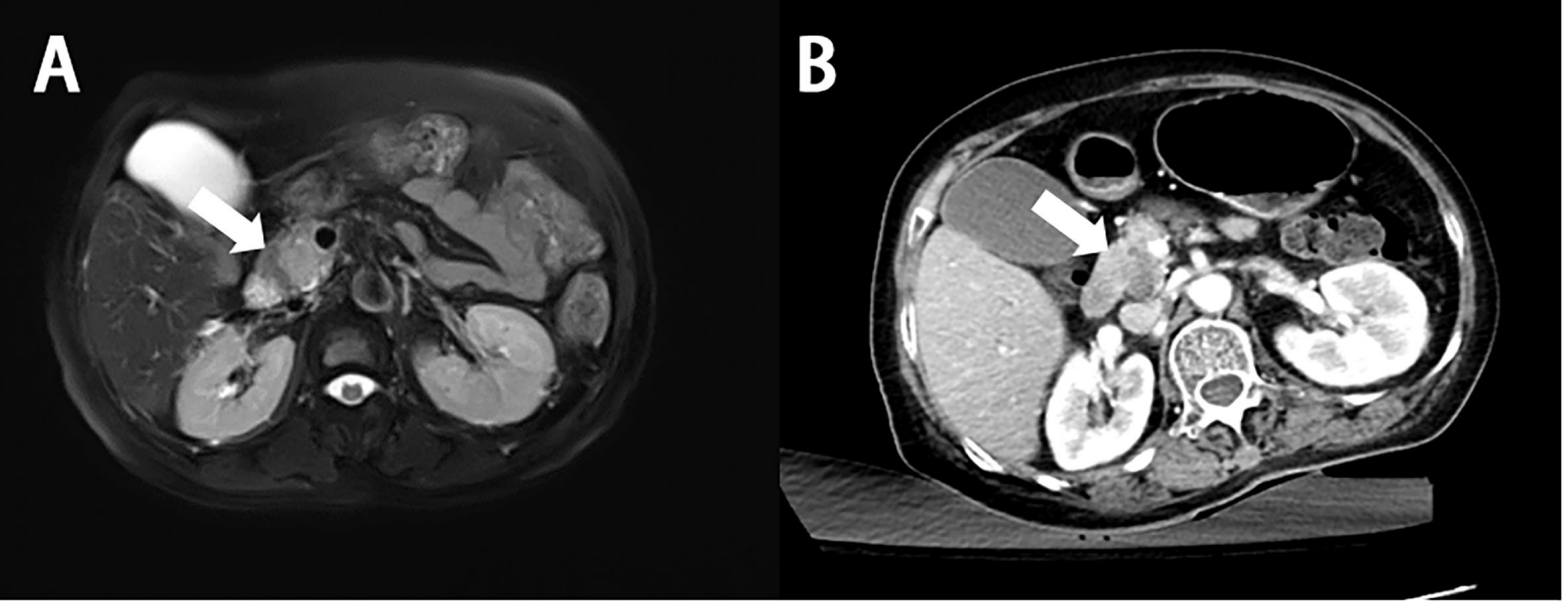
Imaging finding of primary pancreatic diffuse large B-cell lymphoma. (A) MR shows a lesion with hyperintense signal on the T2-weighted images (white arrow). (B) On enhanced CT, the lesion shows a mild enhancement, lower extent than the normal parenchyma (white arrow).
